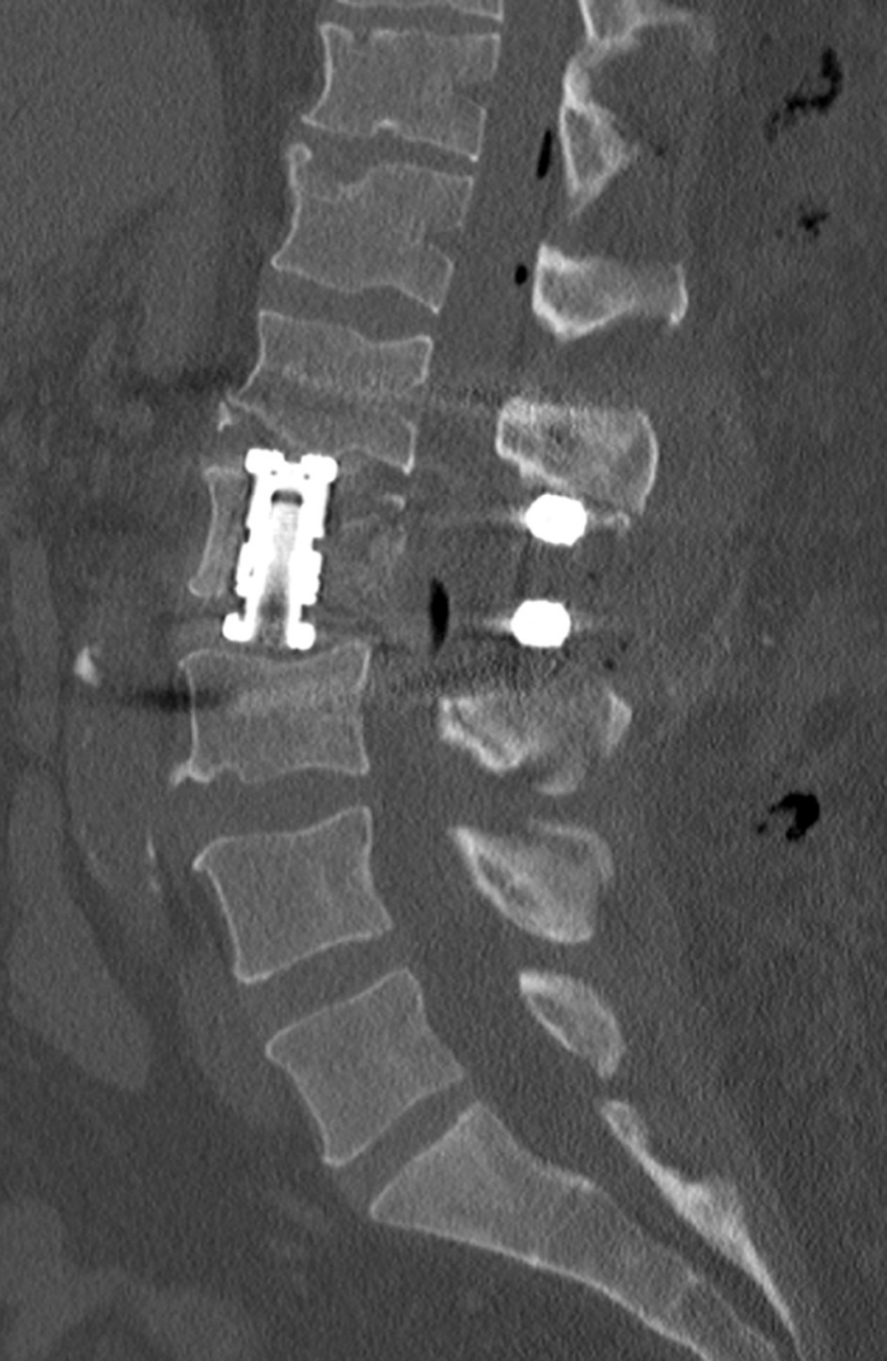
Postoperative CT shows interval corpectomy and placement of expandable cage.
